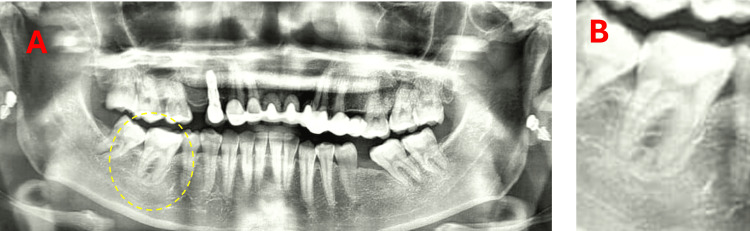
Preoperative radiographic findings. (A) Orthopantomogram showing tooth 46 (circled) with deep recurrent caries and periodontal ligament (PDL) widening. (B) Magnified view of the affected tooth.
